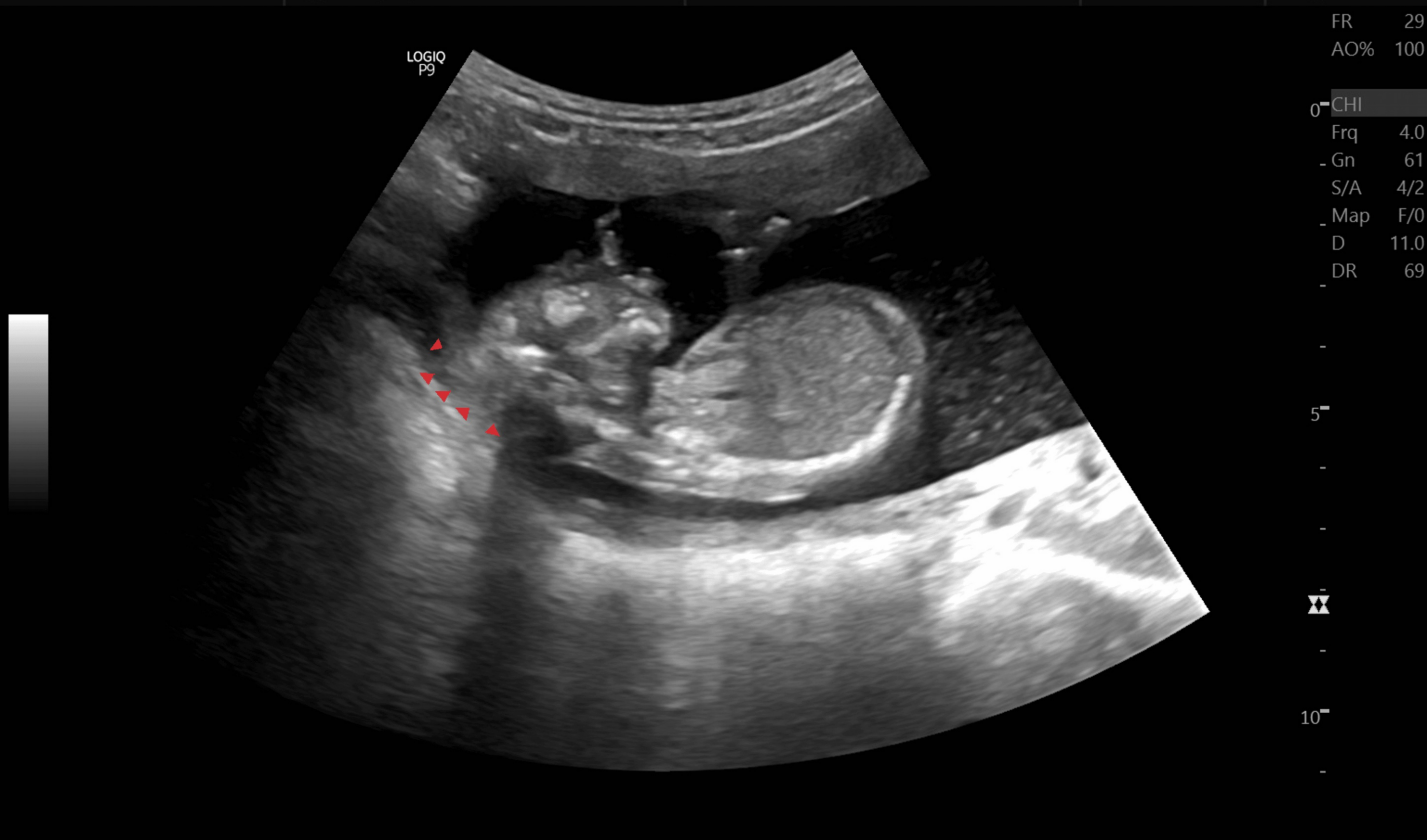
B-mode ultrasonography of the fetus in the mid-sagittal plane. Multiple arrowheads (▶) showing a large amount of angiomatous stroma cephalad to the base of the skull.
